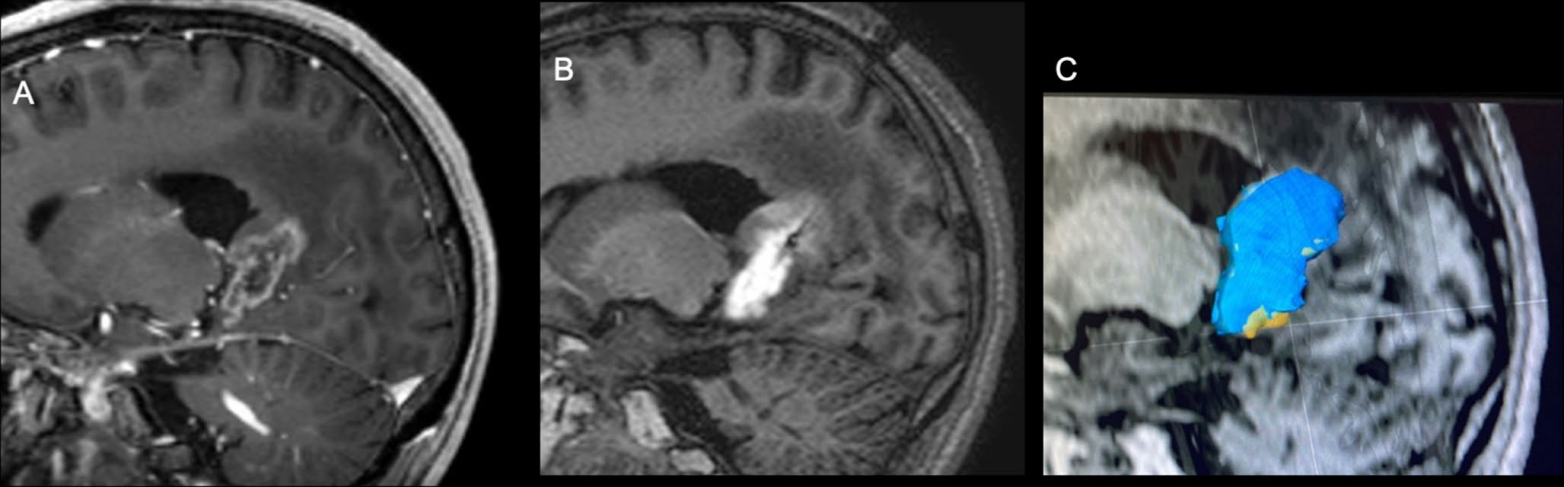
60 year-old patient with left temporal GBM recurrence (CED25) demonstrated by enhancing mass in the left callosal splenium on T1-weighted sagittal MPRAGE image (A). During CED, the area of gadolinium contrast deposition grows to cover 94% of the tumor, as demonstrated on intraoperative T1-weighted sagittal MPRAGE image (B). Volume segmentation of gadolinium infusate administered during CED (blue) overlaid with volume segmentation of enhancing mass (orange) demonstrates extent of coverage
